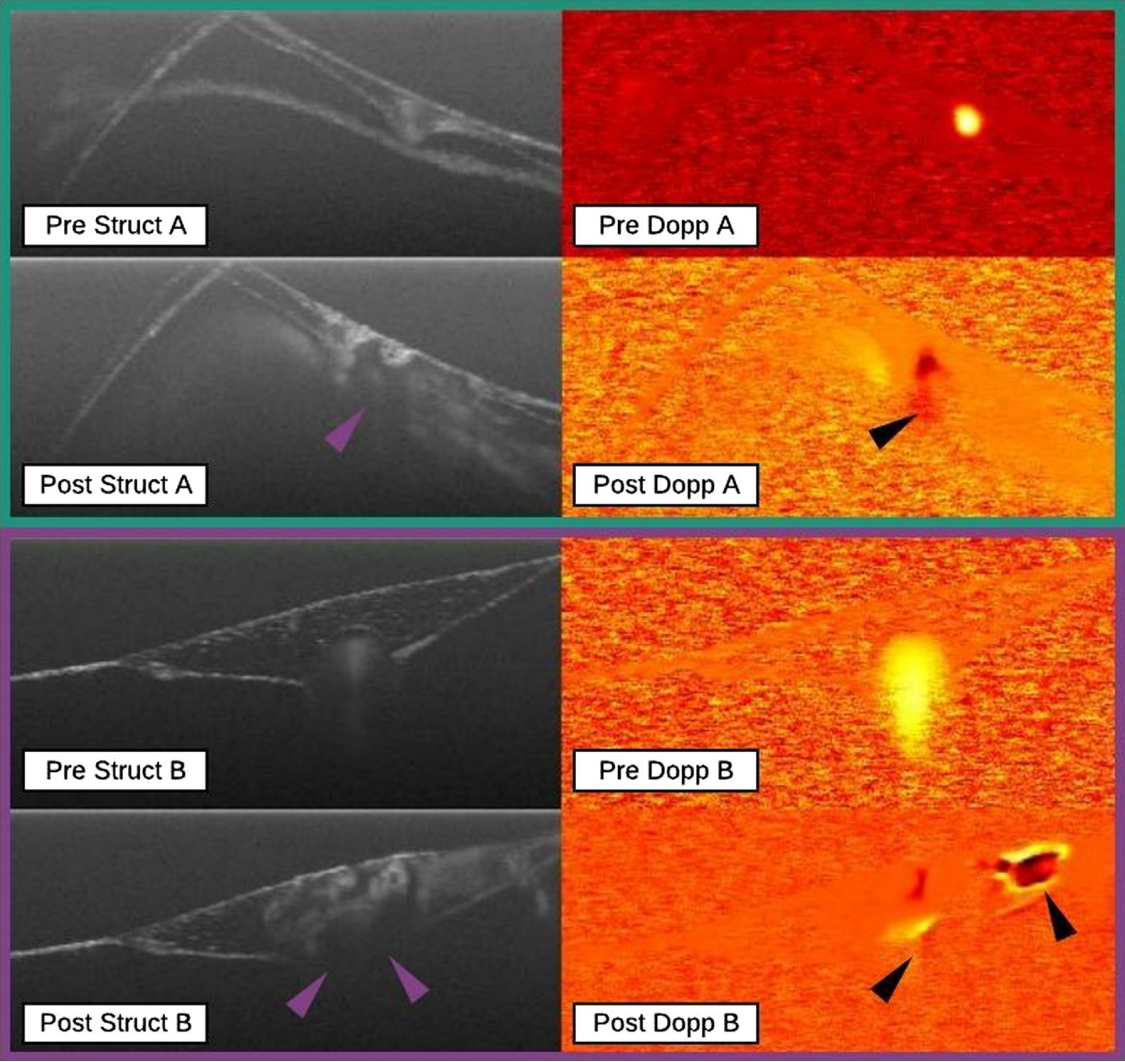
Rupture data points. Both data points A (green rectangle) and B (purple rectangle) demonstrate wall breach indicated by purple arrows while the presence of the diffuse Doppler signal (black arrows) supports the rupture classification. ROIs are left unlabeled for clarity.
